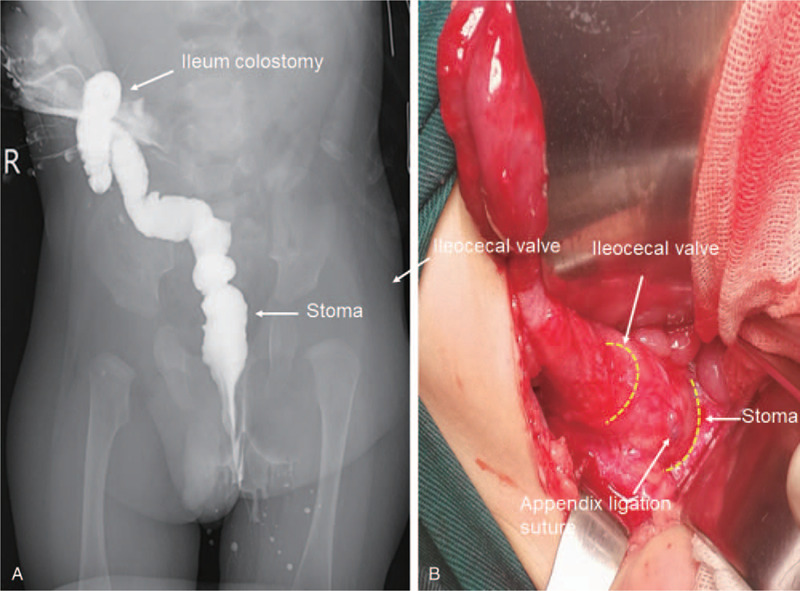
Serial post-magnamosis radiographs of X-ray images and surgical findings. (A) Post-magnamosis contrast enema demonstrating the absence of stricture; (B) Surgical for fistula closing reveals the anastomotic site soft and healing well without stricture (the dotted line is the location of the anastomosis).
